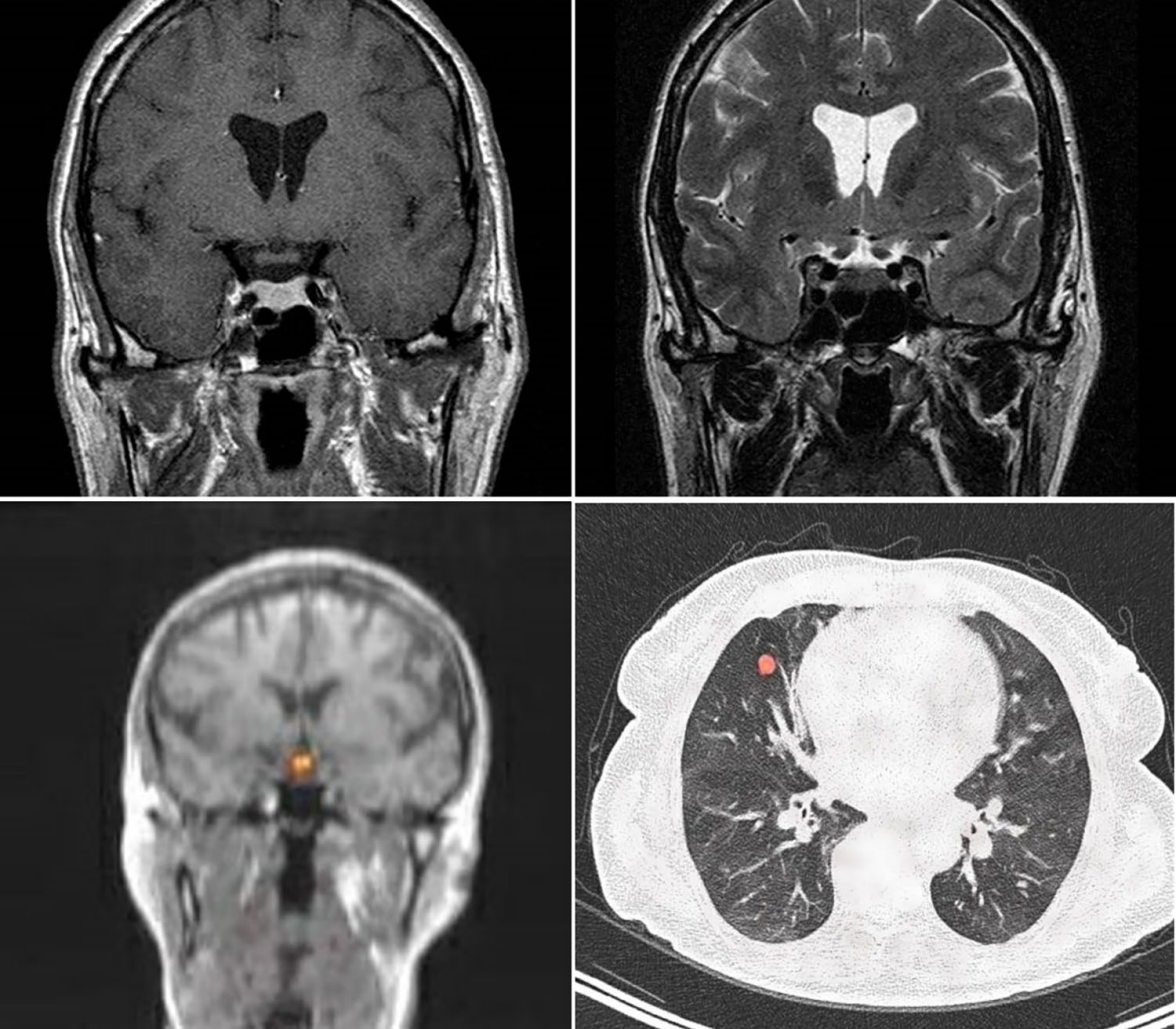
Ectopic acromegaly. 73-year-old woman with acromegaly; serum IGF-1 levels were fivefold x upper limits of normal (ULN), and GHRH levels were threefold ULN. Upper panels, from left to right: T1 weighted + Gadolinium-diethylenetriamine-pentaacetic acid (Gd-DTPA) MRI shows a slight diffuse enlargement of the pituitary; T2W MRI shows a hypointense signal in the pituitary. Lower panels, from left to right: ^68^Ga-DOTATAE PET MRI shows uptake in the pituitary area; ^68^Ga-DOTATE PET CT shows uptake in an SSTR-positive bronchial NET in the right middle lobe. Final diagnosis was bronchial NET with ectopic GHRH production [77]
